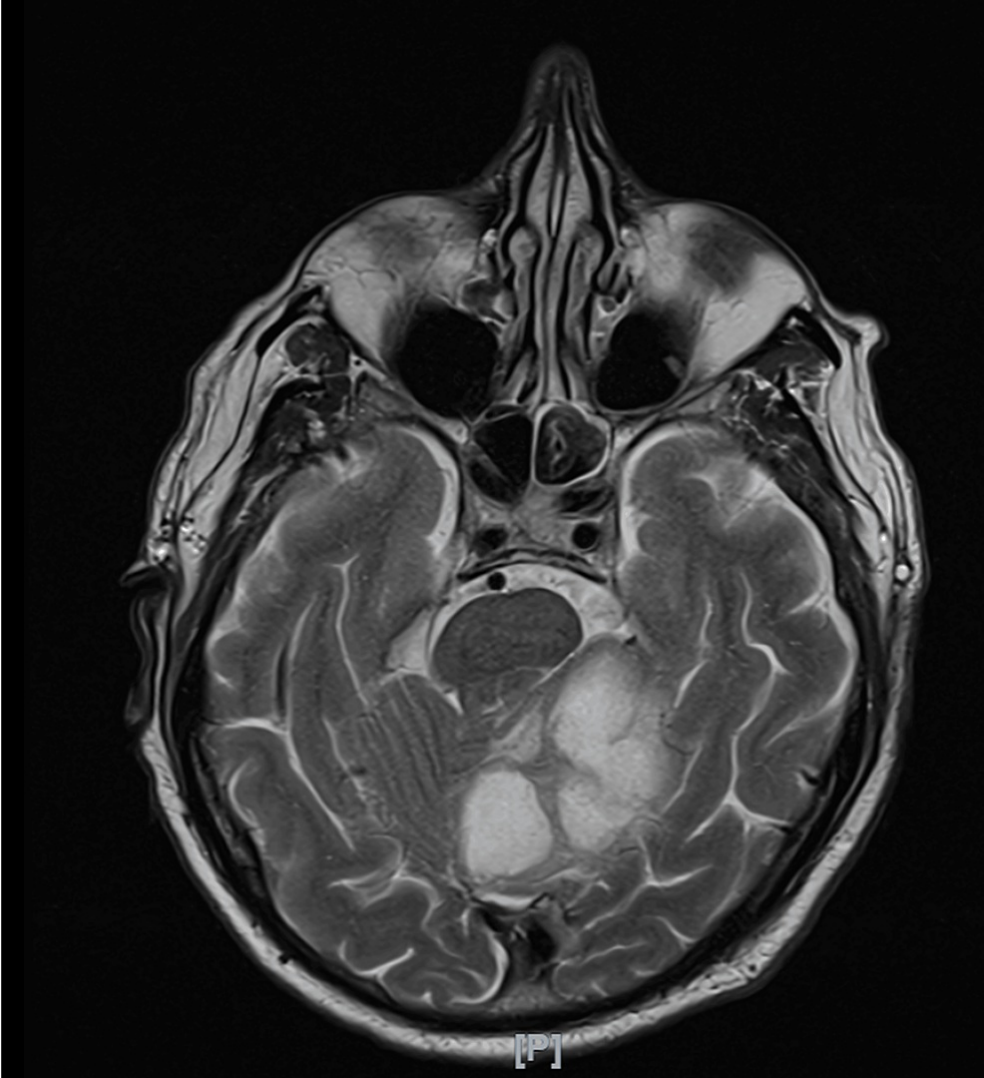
Results of the MRI of the brain in August 2023, revealing discernible lesions located in the cerebellum and left parietal lobe.
